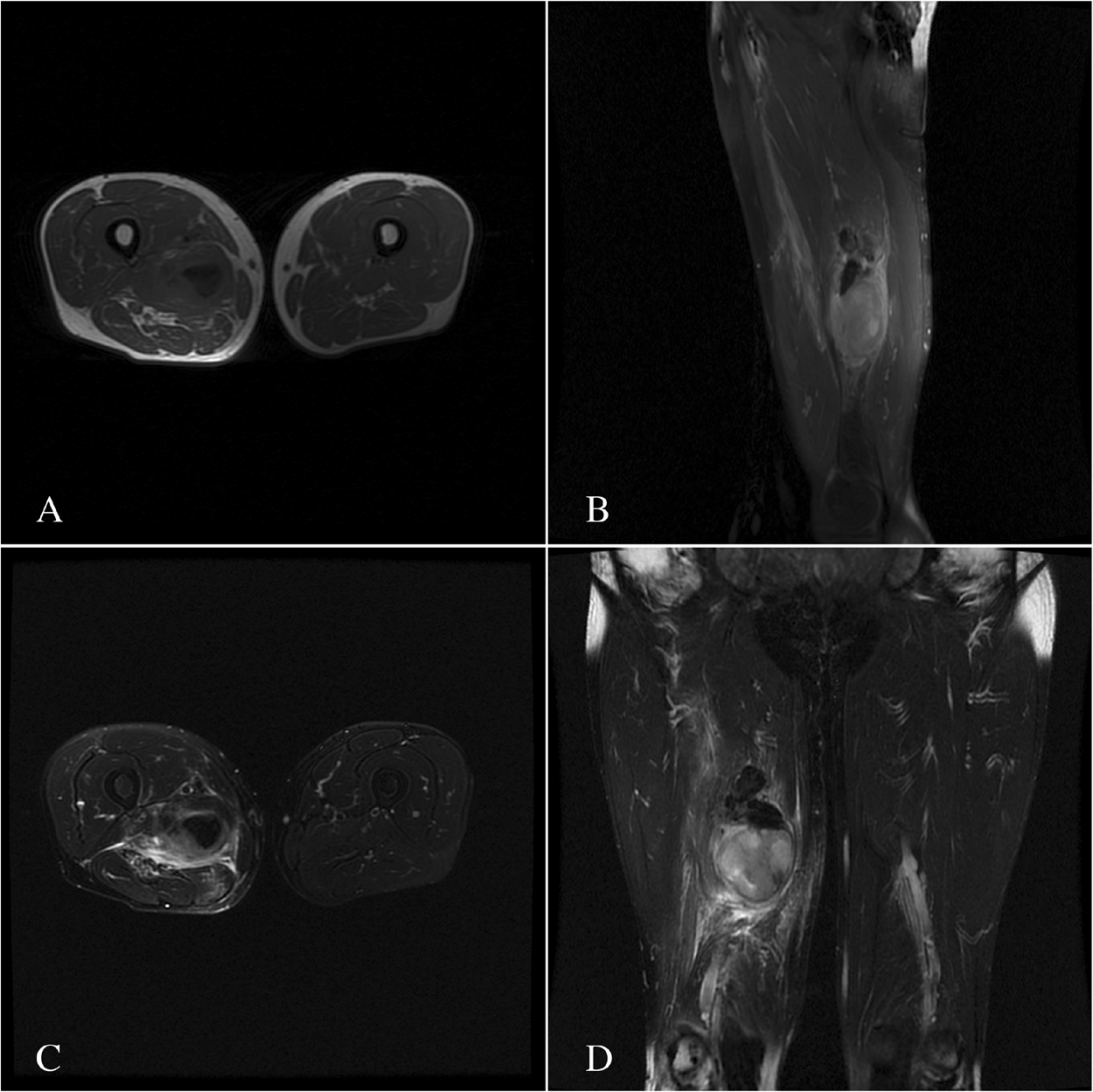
T1-weighted and STIR images in MRI for the lesion. Axial and sagittal T1-weighted image (A, B) show an inhomogeneously isointense mass in the posterior thigh. The lesion demonstrates inhomogeneously hyperintense on axial and coronal STIR images (C, D).
